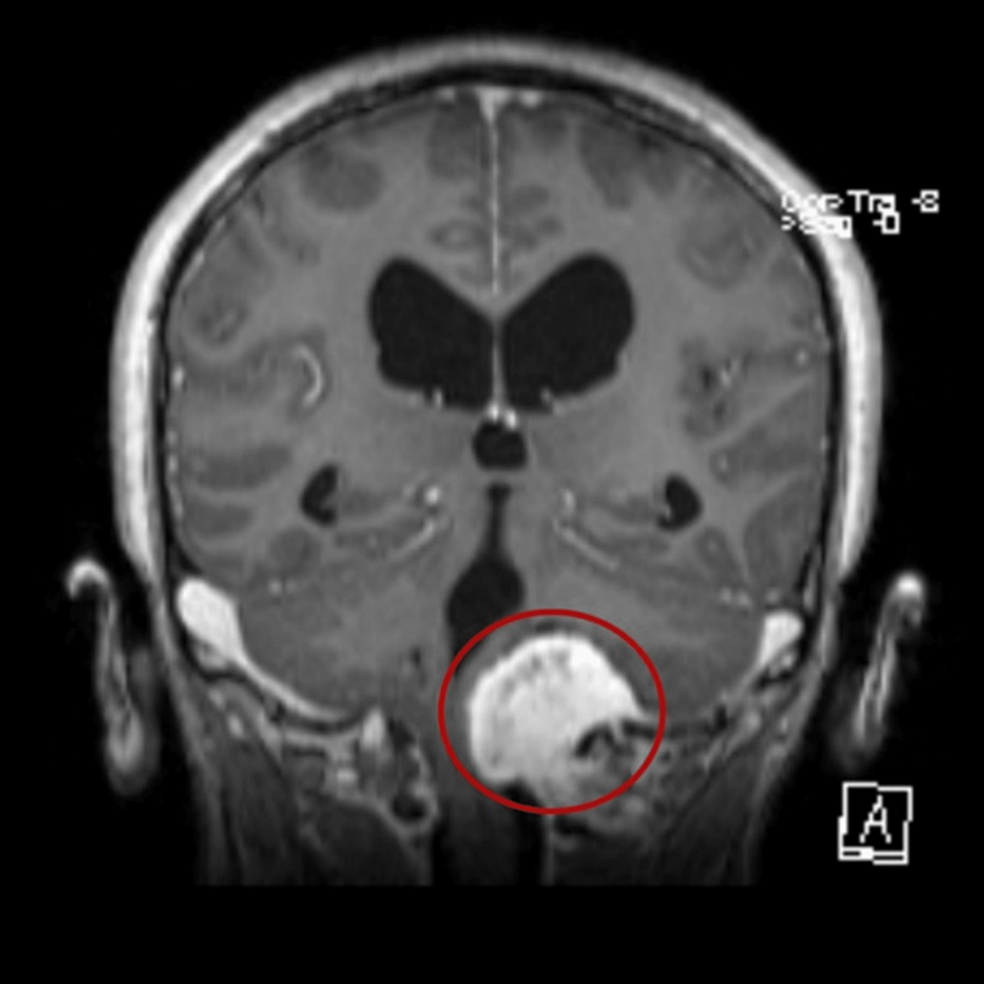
Cranioencephalic MRI revealing a spinoclival tumour with 36 mm in its bigger axis (red circle). MRI: magnetic resonance imaging.
